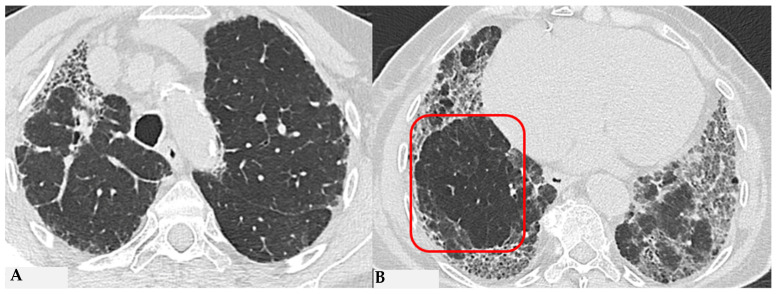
Axial chest HRCT scan. Asymmetric fibrotic alterations with volume loss on the right in the upper lobe (A); in the lower lobes on the right, a significant extensive area of reduced attenuation is evident, suggestive of air trapping (red box), with components of distorted GGO and fibrosing characteristics with intra- and interlobular thickening and traction bronchiectasis/bronchiolectasis (B).
